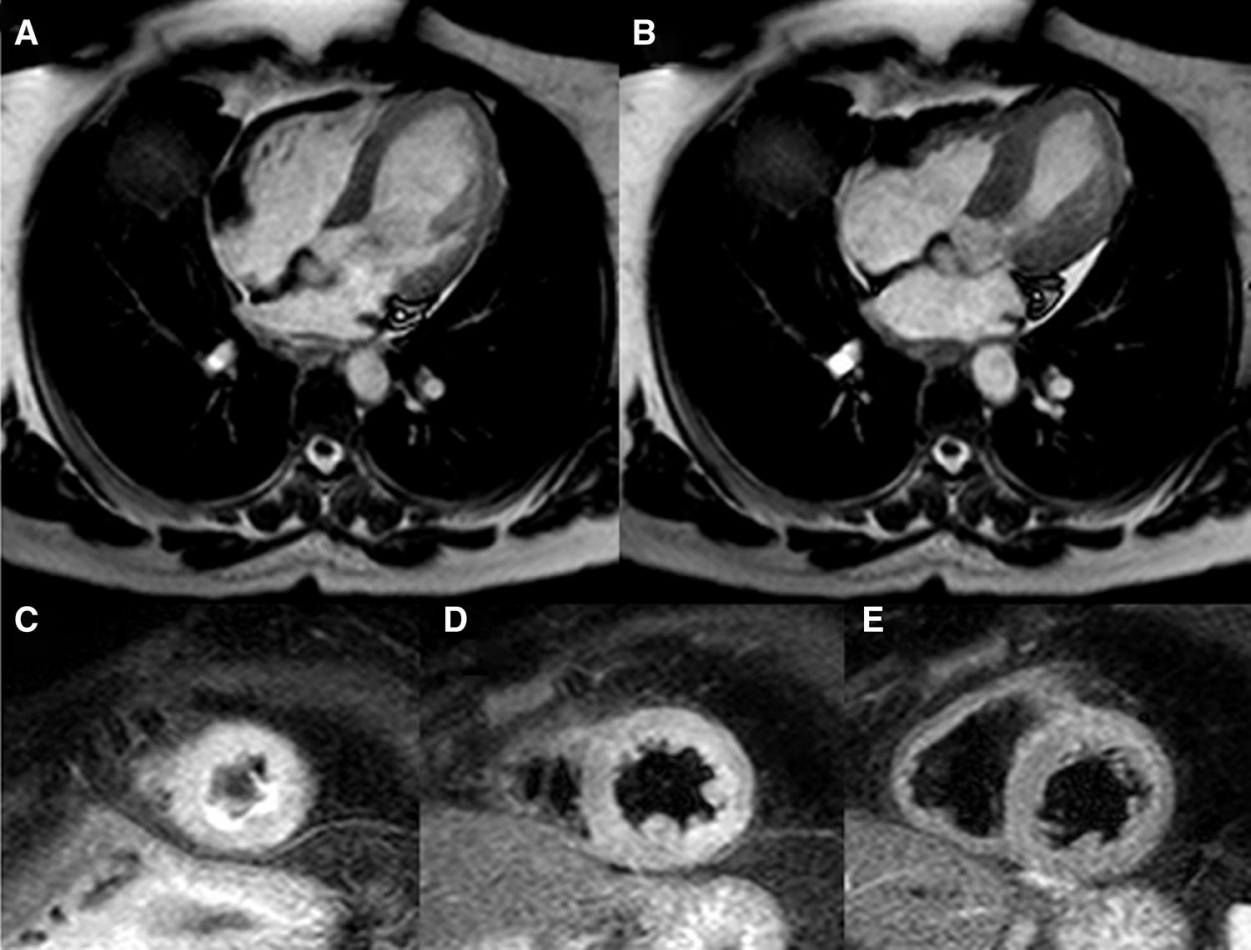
Myocardial oedema in takotsubo syndrome (TTS). TTS patient with typical apical ballooning displayed in end-diastolic (a) and end-systolic (b) bSSFP imaging in horizontal long-axis view. T2-weighted imaging (short tau inversion recovery) demonstrates circumferential myocardial oedema in the left ventricular apical (c) and mid-ventricular (d) segments without involvement of the basal segments (e). bSSFP, balanced steady-state free precession
